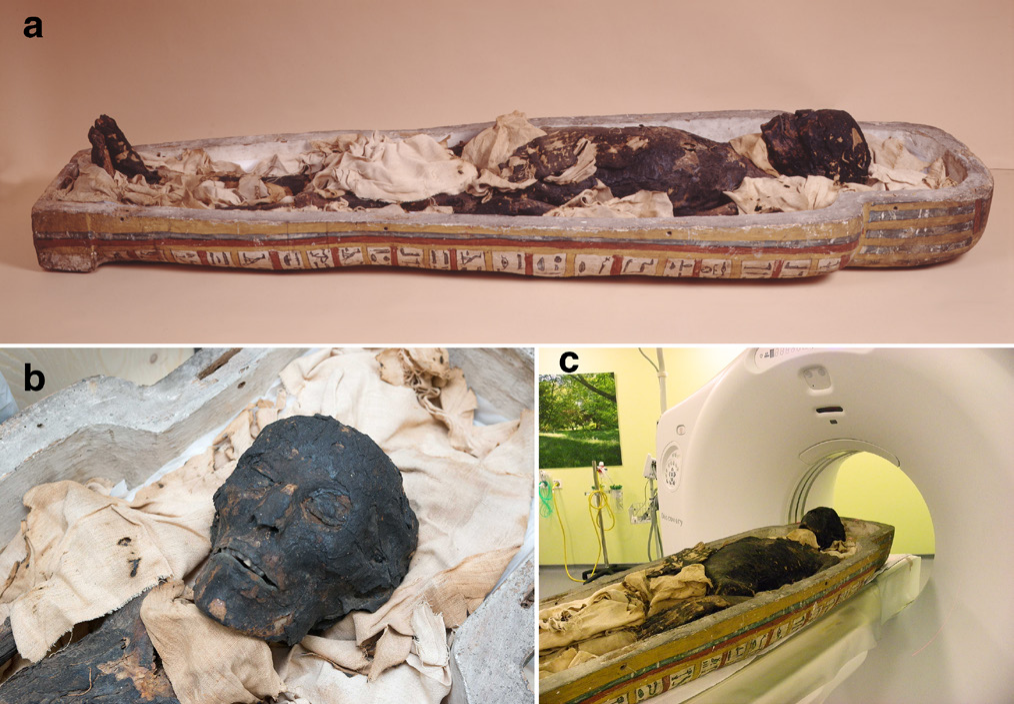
(a) Ta Kush in the inner wooden coffin. (b) Ta Kush uncovered face. (c) Ta Kush in the CT scanner.
